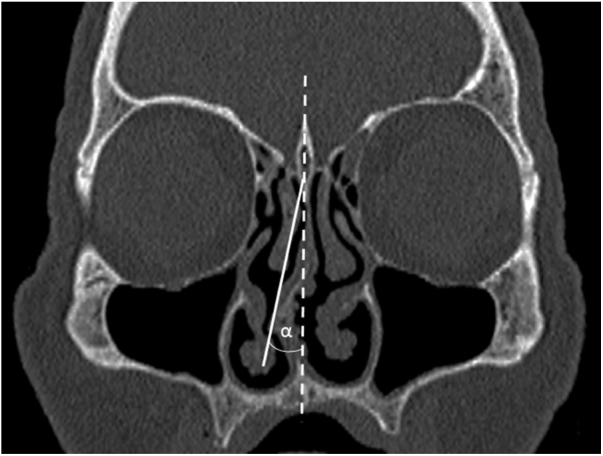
Measurement of the NSD angle on coronal CT image.
